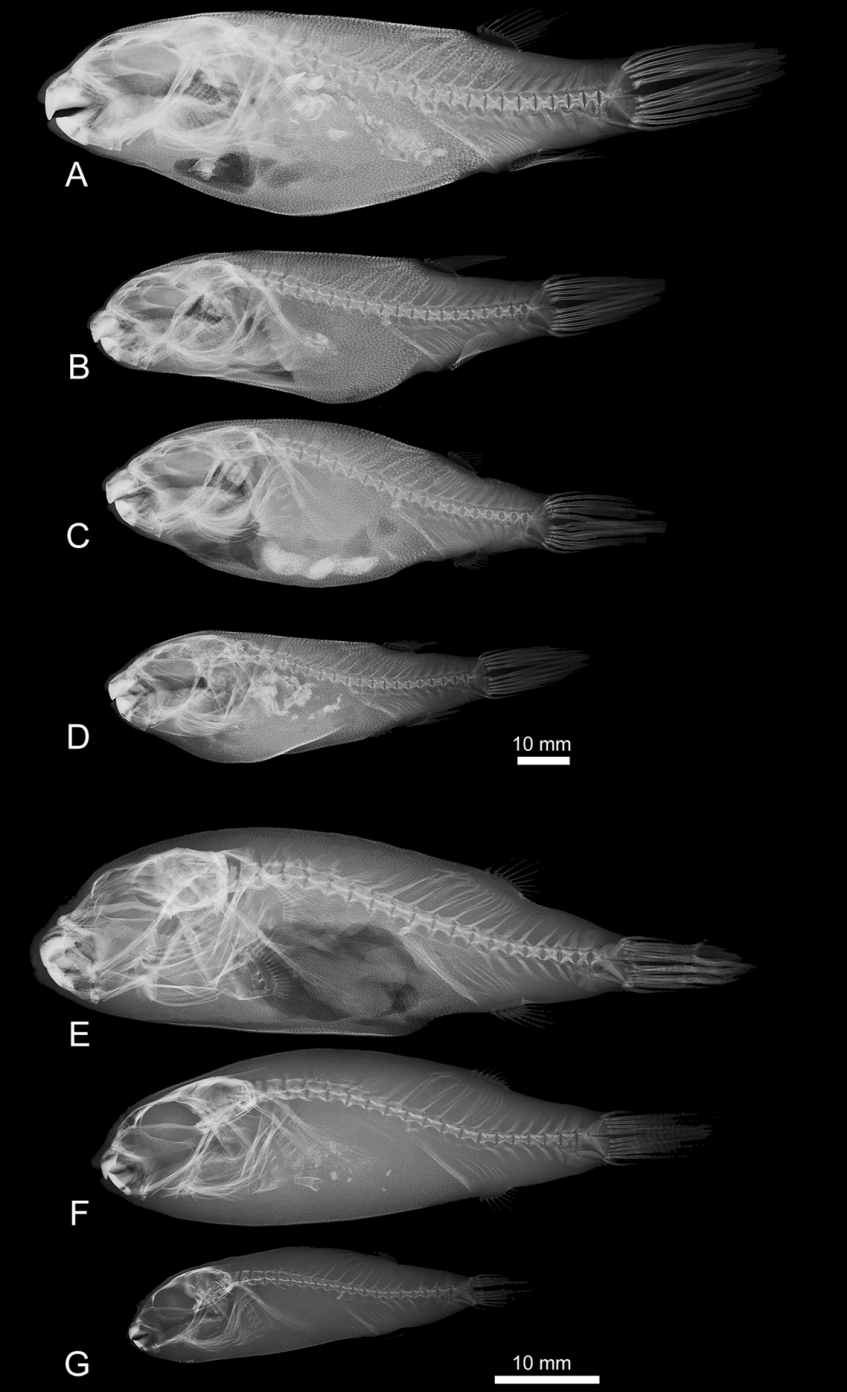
High-definition x-ray images of: A–D, *Colomesus psittacus* USNM.393077; E–G, *Colomesus asellus* USNM.191569. Scale bar equals 10
